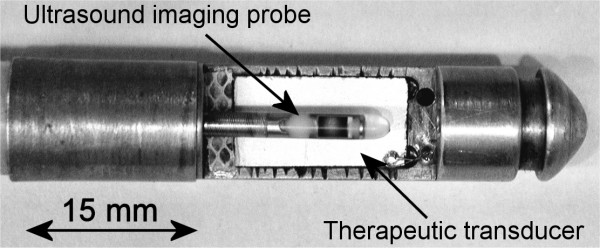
Head of the intraluminal high intensity ultrasound applicator. The ultrasound imaging probe is in front of the therapeutic transducer to image the region of interest.
